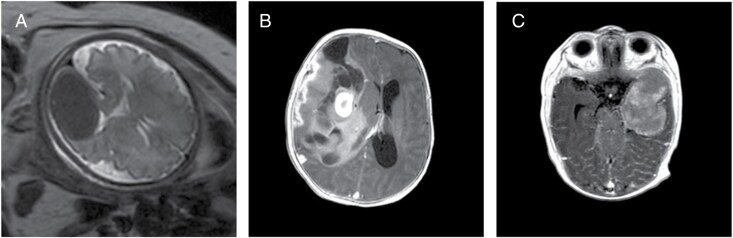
(A) T2-weighted fetal MRI showing hemispheric solid mass in the occipital lobe; (B and С) T1-weighted MRI with contrast enhancement of cystic-solid (Rӧ-subtype 1, B) and solid (Rӧ-subtype 2, C) IHG.
